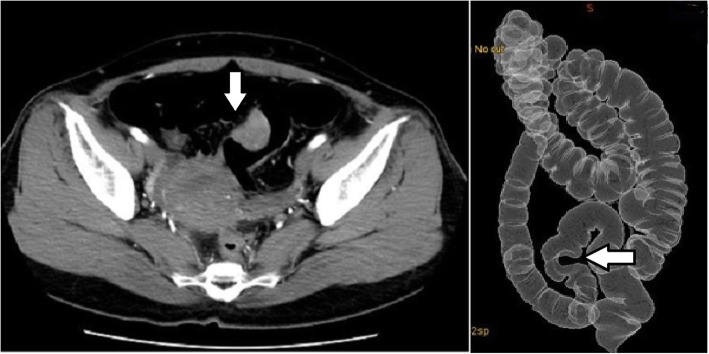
Computed tomography (CT) reveals the thickened wall of the sigmoid colon (arrow), and CT colonography shows the deformed wall in the sigmoid colon (arrow)
